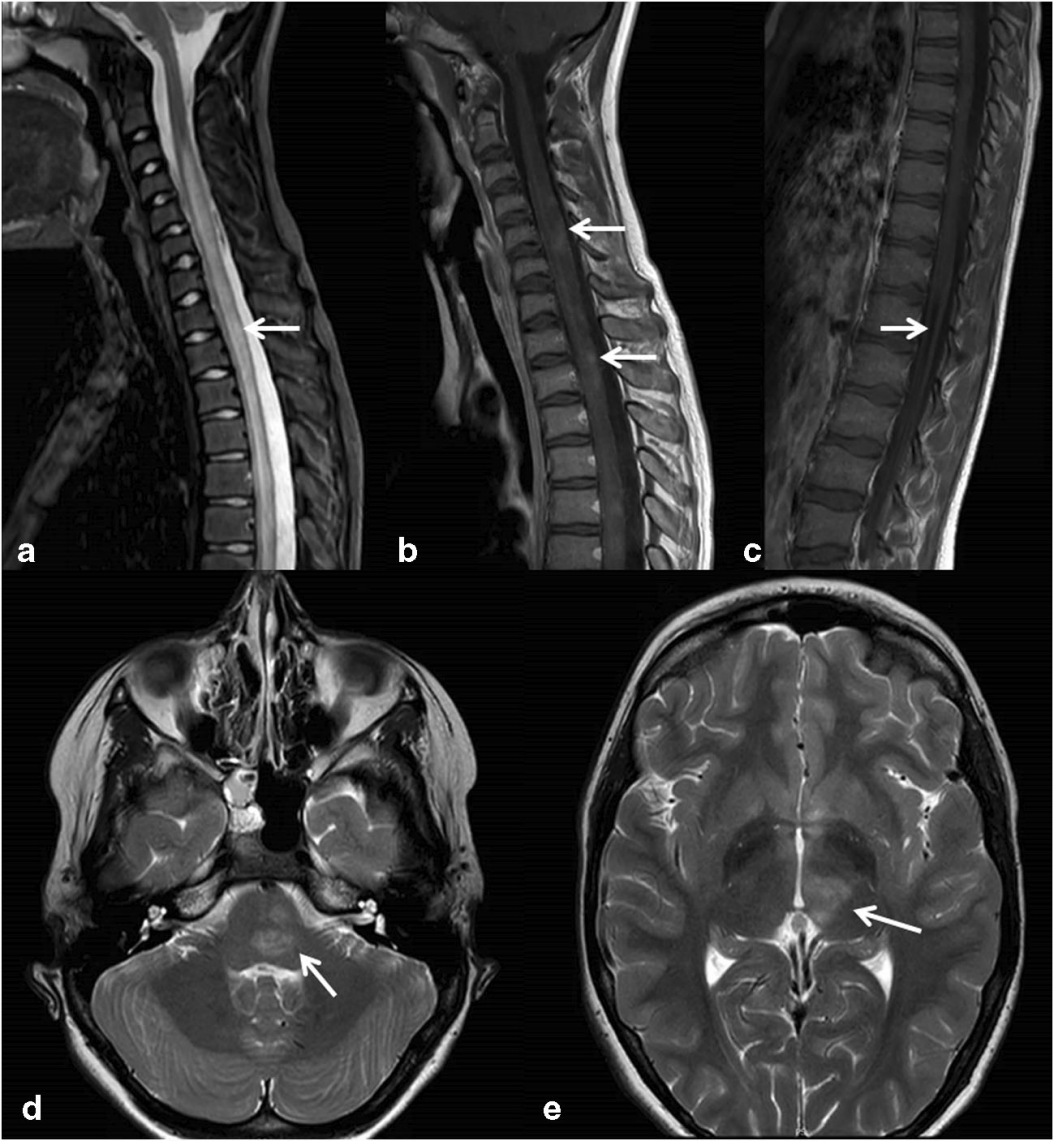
MRI of a 13-year-old boy with a provisional diagnosis of acute demyelinating encephalomyelitis. a Sagittal short tau inversion recovery (STIR) with edematous cervicothoracic spinal cord from the level of C4. b Sagittal T1 of the spinal cord showing diffuse areas of slight enhancement. c Enhancement of mainly dorsal roots in a sagittal T1 of the lumbar spine. d and e Transverse T2 at the level of the pons (d) and thalamus (e) showing asymmetric hyperintense areas.
